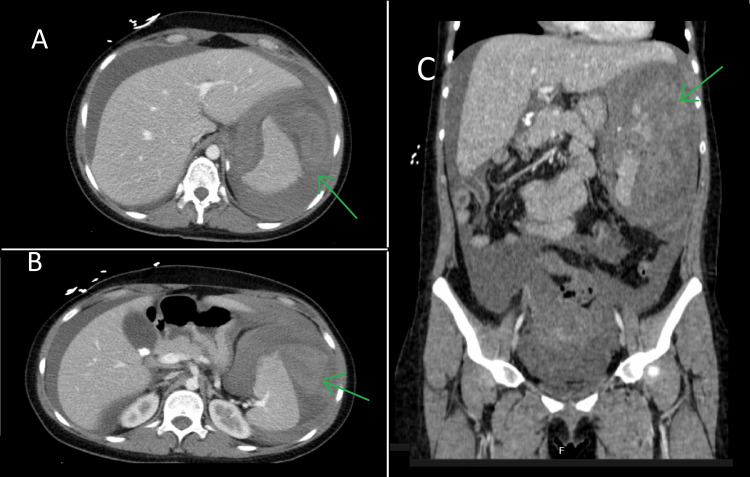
(A and B) Axial and (C) coronal section of an abdominal CT. It can be seen the presence of contrast material extravasation, along with the detection of free peritoneal fluid and hyperdense elements within the LUQ. CT: computed tomography.
